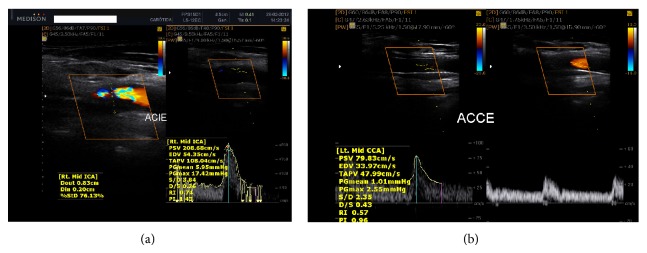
Ultrasonography of the carotid arteries showing the presence of plaques in the left internal carotid artery (a) and left internal carotid artery after stent implantation (b).
